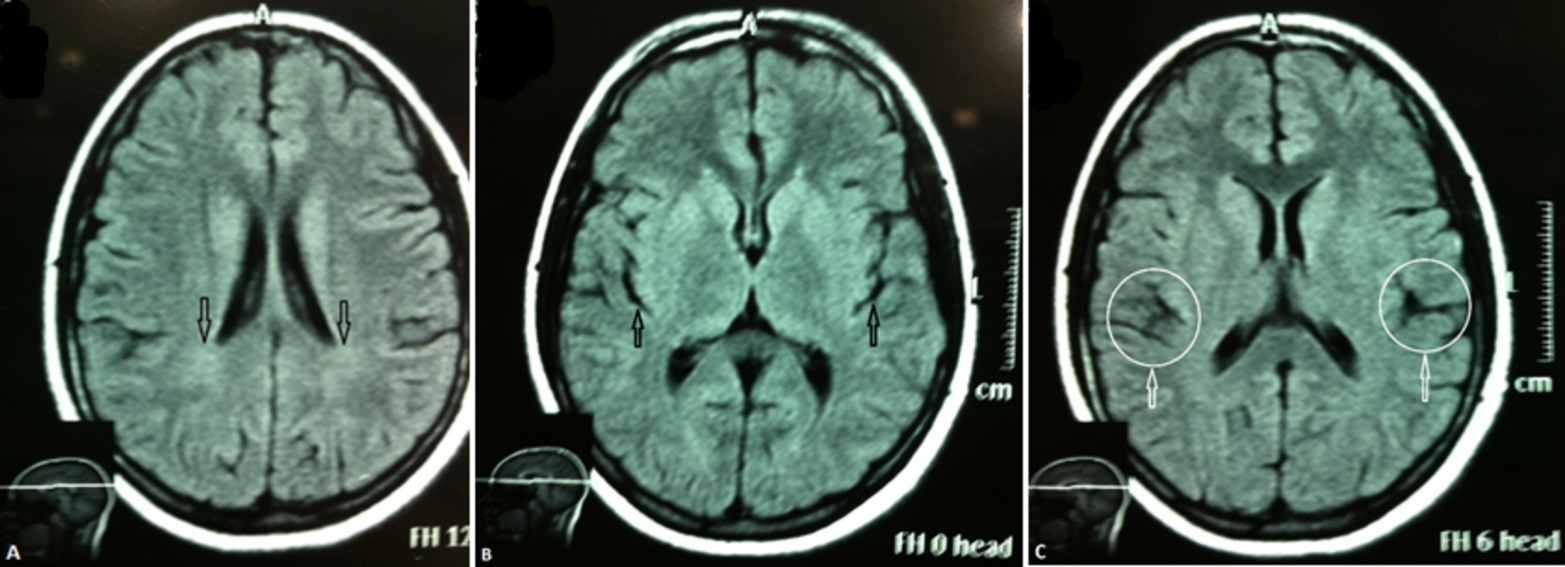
MRI brain findings Magnetic resonance imaging (MRI) showing moderate hyperintensity in the parietooccipital region bilaterally (arrows in A). Cortical atrophy is present, signified by markedly enlarged sulci (arrows in B). Enlarged sulci representing cortical atrophy signified by encircled areas in C.
